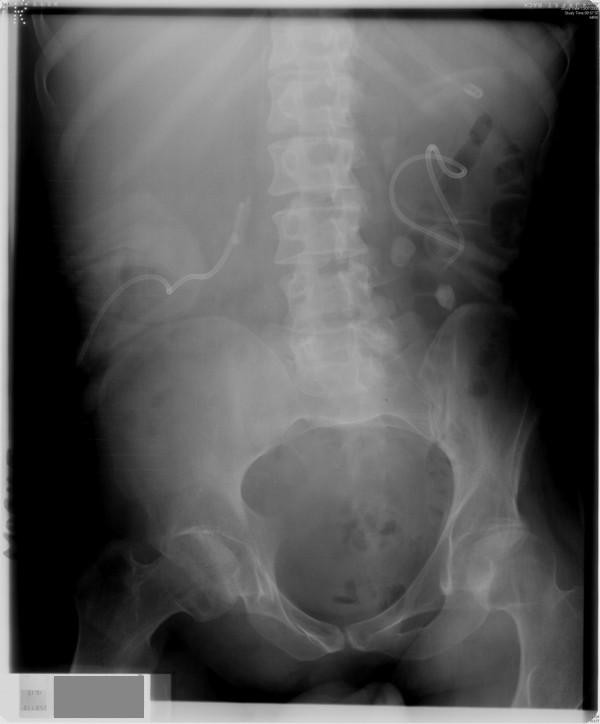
X-ray of kidneys (13 July 2005) showed nephrostomy catheters in both kidneys. Calculi were present in left renal pelvis and inferior calyx.
